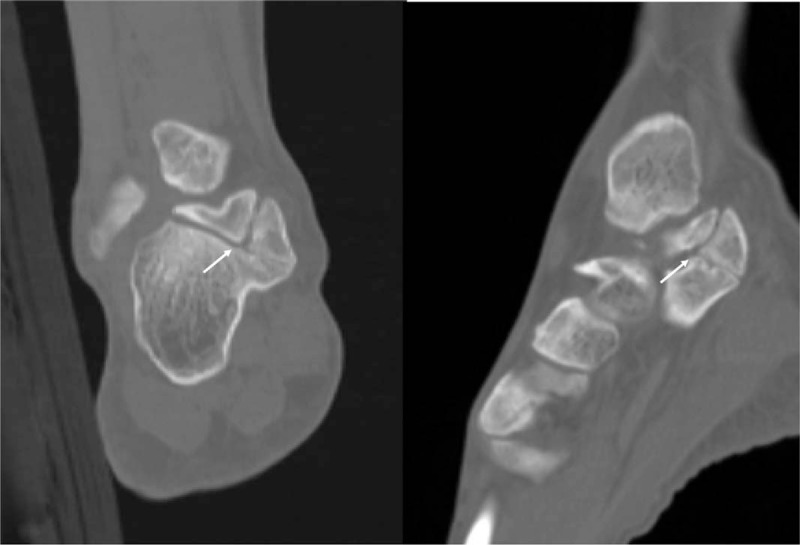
Computed tomography (CT) scan of the ossicle. Oblique coronal CT (A) and sagittal CT cuts showing the appearance of the ossicle and position of the accessory ossicle articulating between the talus and calcaneus. The accessory ossicle is united with the sustentaculum tali and a narrow and irregular interface (arrow) between the accessory ossicle and adjacent bones is noted. CT = computed tomography.
